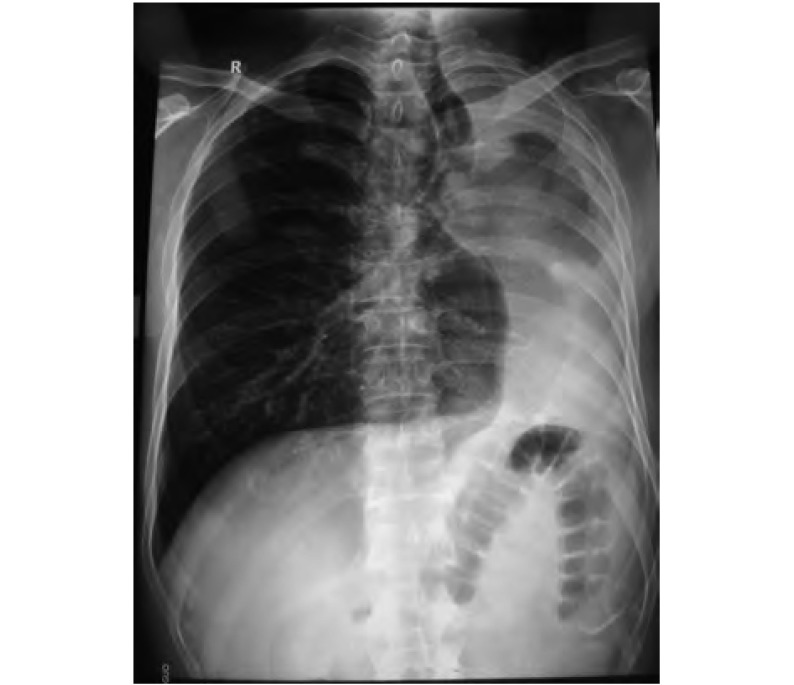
术前胸片示气管腔内见肿块影，左主支气管未见，左肺不张，右肺过度扩张 X-ray before operation showed mass in trachea, the left bronchea wasn't showed, left lung collapsed, and right lung over expanded
